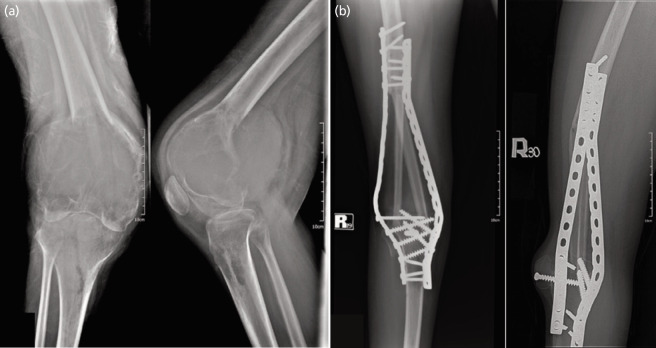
KRA with dual plates in a 24/F. (a) Pre-operative radiographs show a distal femur giant cell tumour with soft tissue extension. Distal femur resection and KRA were performed. (b) The 3.5 years post-operatively show good union with fibular grafts proximally and distally.
